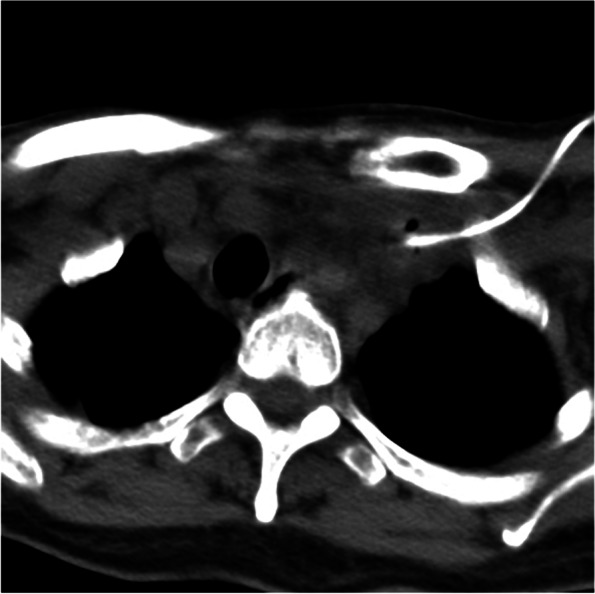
CT scanning detected the PICC in the left subclavian vein and left internal jugular vein with thrombus formation. A few gas bubbles were seen along the left internal jugular vein Excessive exudation and inflammatory changes were seen in the interstitial space around the venous thrombus.
